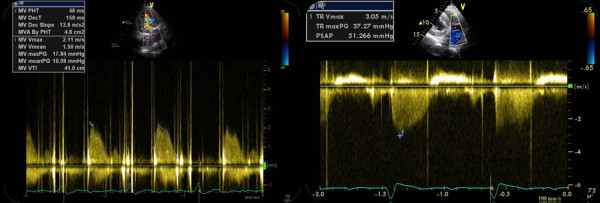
Postoperative transthoracic ecocardiogram showing an abnormally high gradient of the mitral prosthesis (maximal 18 mmHg and mean 10 mmHg), with a deceleration time (< 130 ms), possibly correlated with an increased flow across the prothesis (left panel). High pulmonary artery systolic pressure was assessed (51 mmHg) (right panel).
